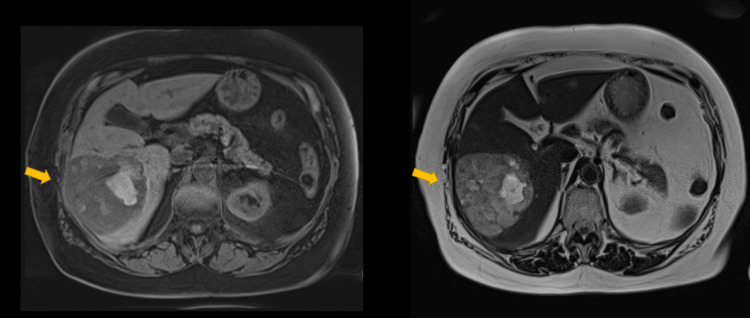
HA mimicking cavernous haemangioma MRI on T1 (left) and T2 (right) sequences. The lesion (yellow arrow) appears hypointense in T1 and hyperintense in T2, interpreted as cavernous haemangioma. MRI: magnetic resonance imaging; HA: hepatic angiosarcoma
